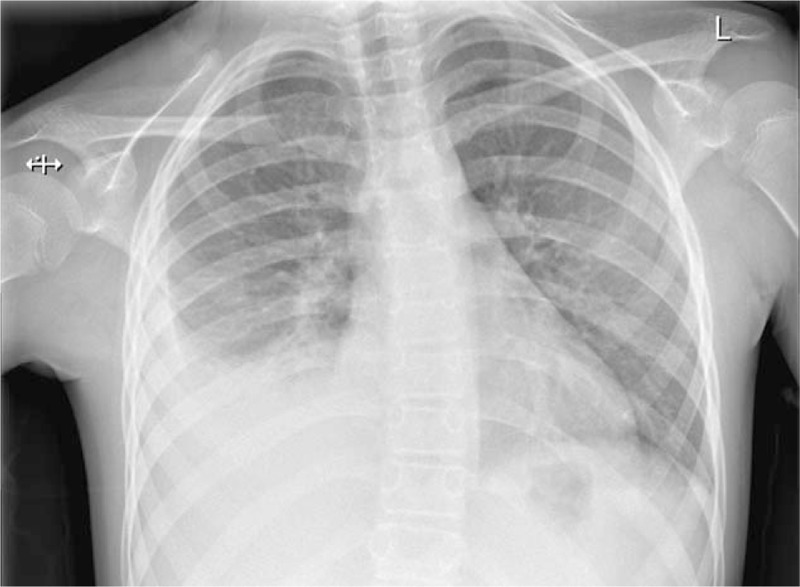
Chest radiograph on day 3 of hospital admission showing a moderate right sided pleural effusion. Note the scoliosis, with the convexity of the spine pointing away from the side of the effusion.
